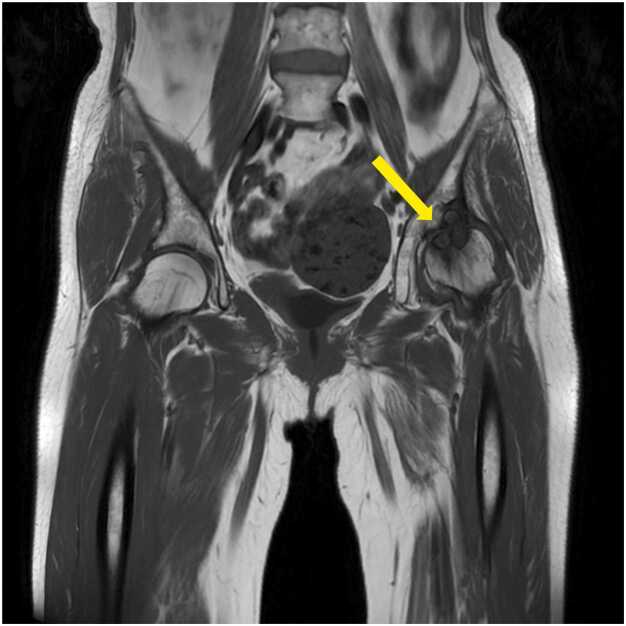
Magnetic resonance imaging (MRI) of the left hip. An MRI scan at the time of presentation revealed a low-signal area on T2 weighted images of the left femoral head and labrum, suggesting cystic changes. These findings are consistent with the osteoarthritic changes caused by congenital hip dislocations.
